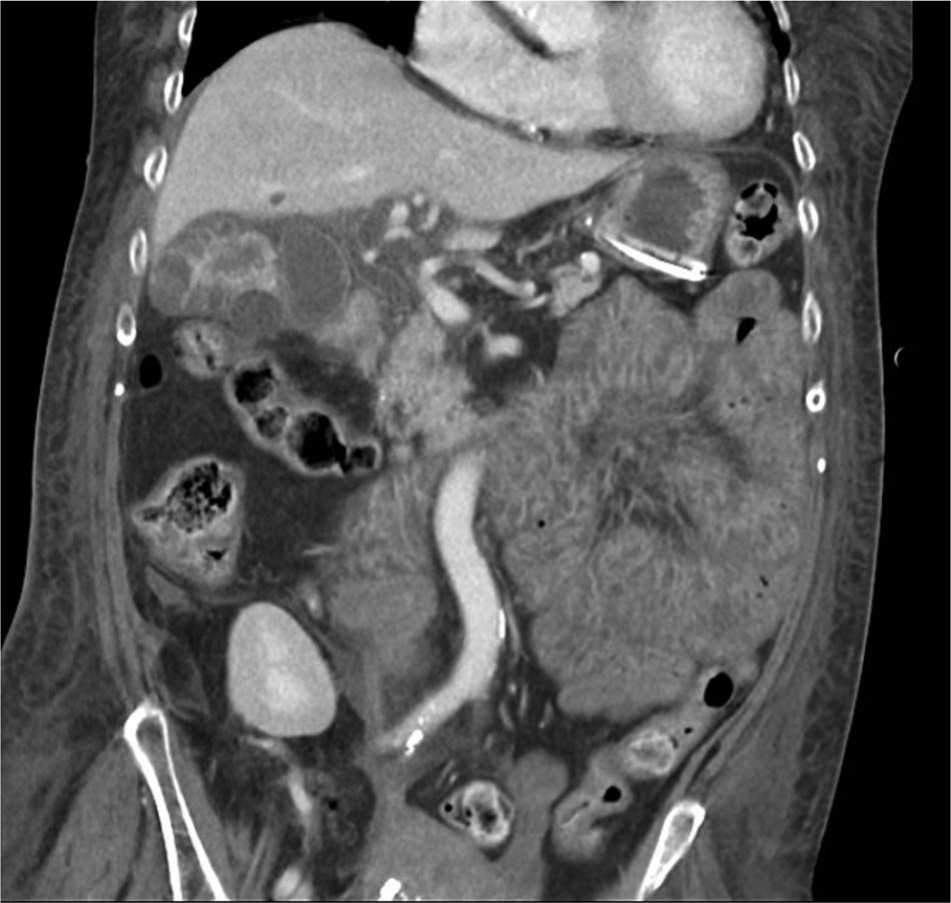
CT chest abdomen pelvis with IV contrast coronal view. The common bile duct is visible and without dilation, note multiple intramural hyperattenuating nodules and gallbladder wall hyperenhancement. This image also shows diffusely edematous jejunum indicative of systemic hypoperfusion.
